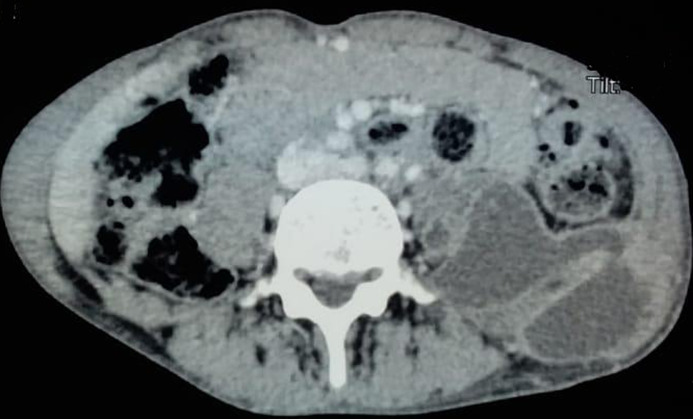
abdomino-pelvic CT showing collections in the left iliac psoas muscle and the left gluteal and the trochanteric region
